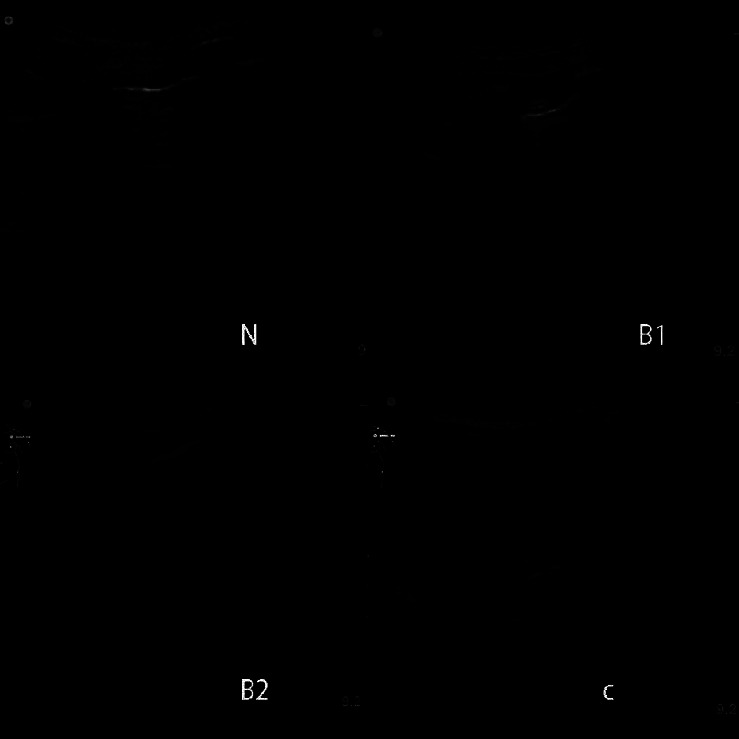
Different lung ultrasound aeration patterns. *Note*. Normal (N, score = 0), moderate (B1, score = 1), severe (B2, score = 2), and consolidation (C, score = 3).
